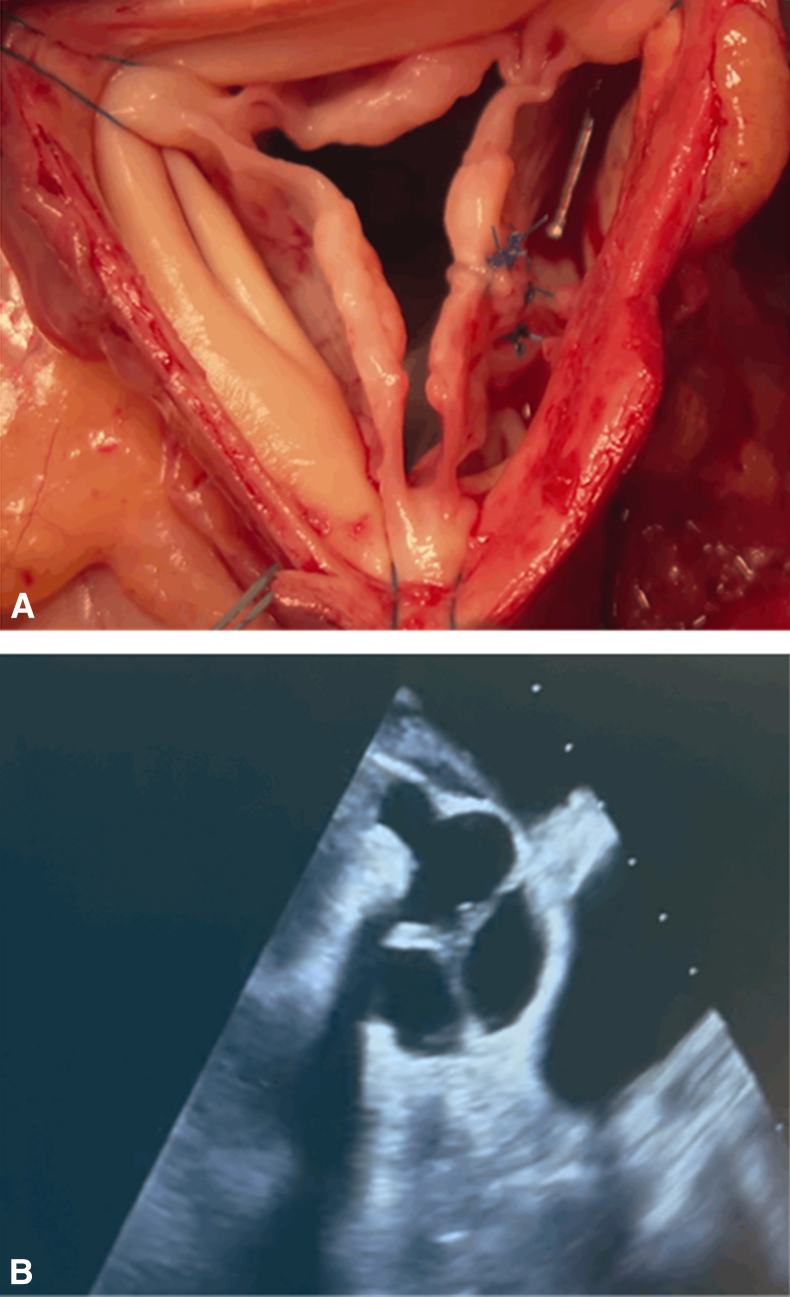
Postoperative surgical (A) and transthoracic echocardiography (B) view.
